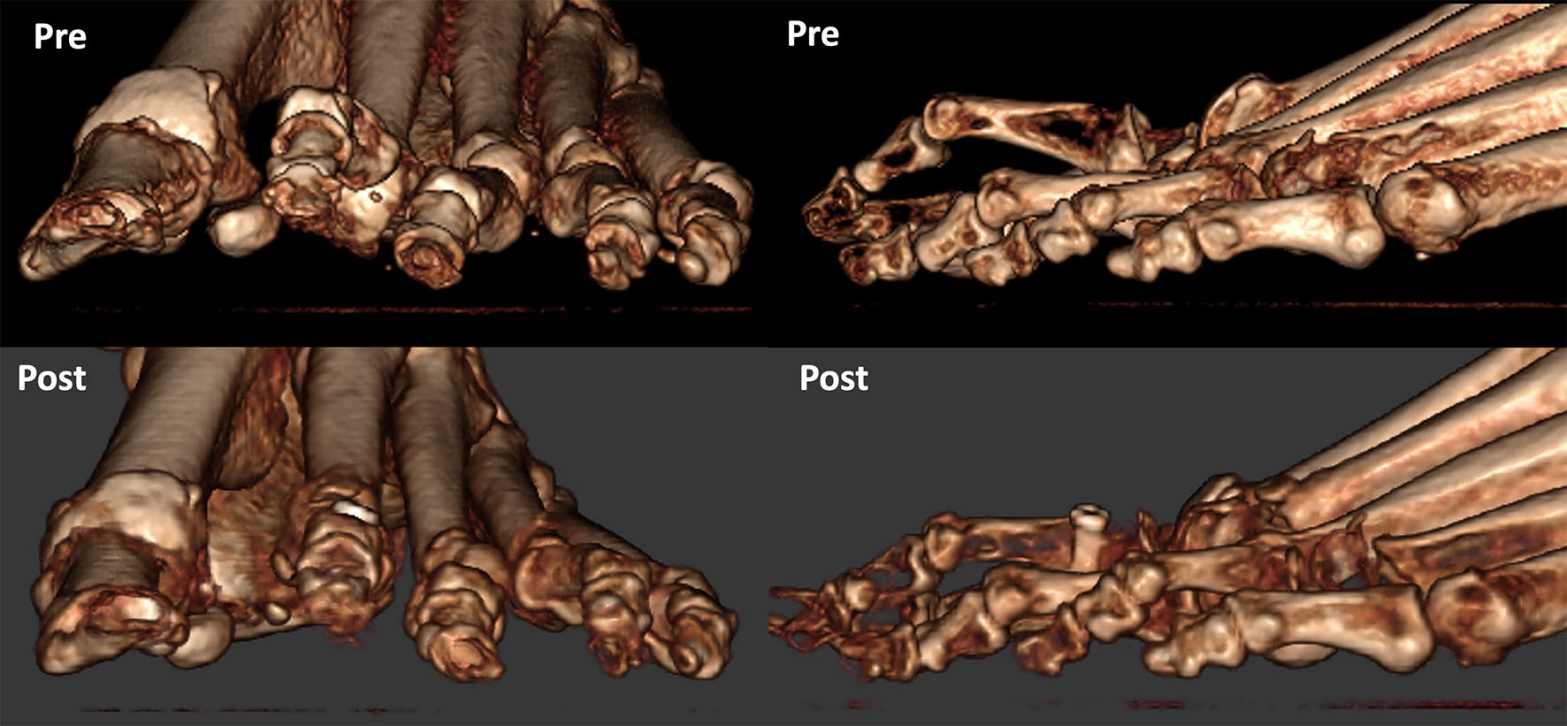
Tree-dimensional surface bone anatomy weight bearing CT reconstruction views of patient’s foot, demonstrating preoperative lesser 2nd toe deformity, and considerable correction 3-months postoperatively
